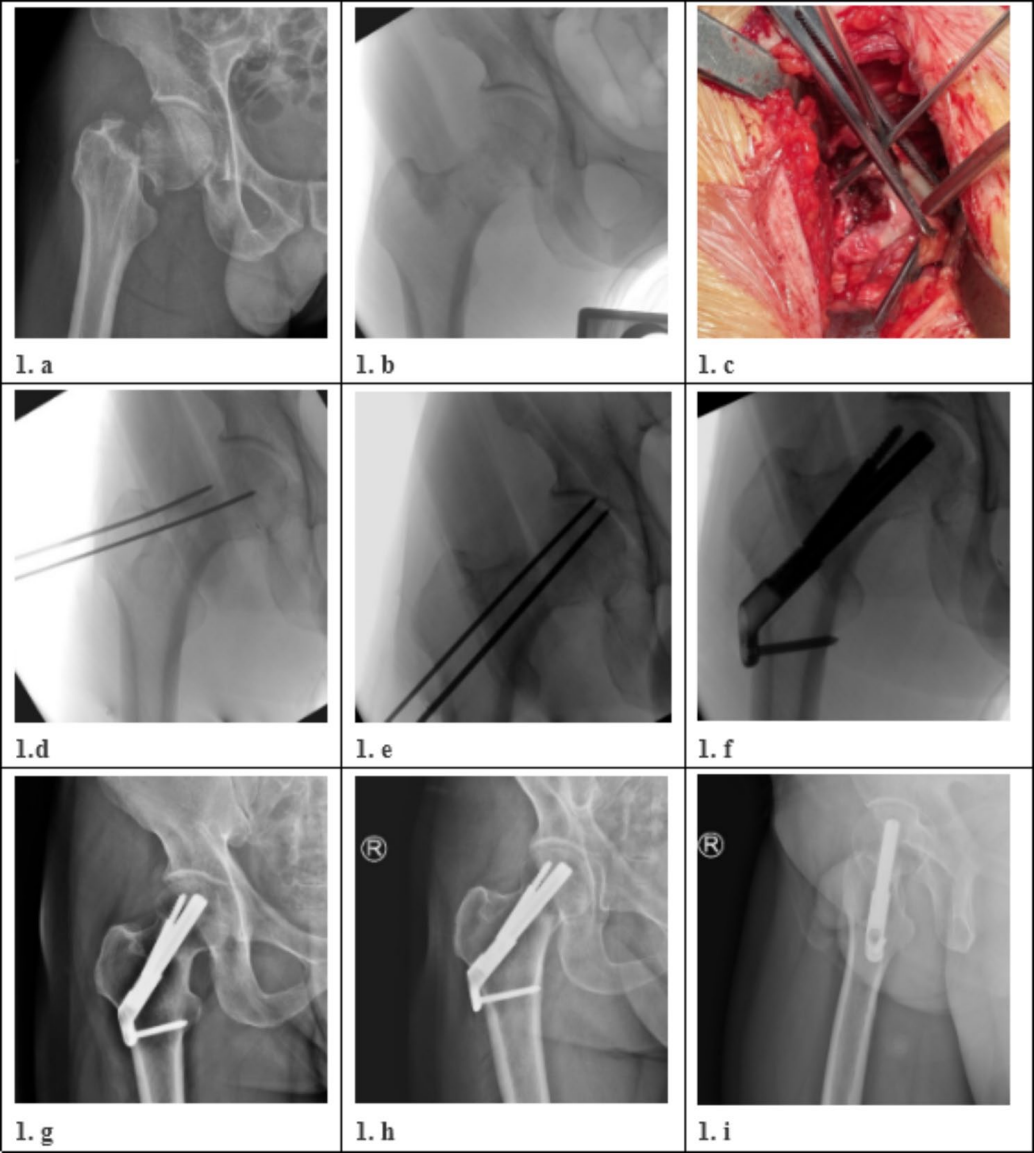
Patient1, Male, fall injury, (1a: Right femoral neck fracture on preoperative X-ray, Garden type IV; 1b: Intraoperative radiography showed that closed reduction could not achieve satisfactory reduction; 1c: Intraoperative incisions image showed that Kirschner wires were inserted into both distal and proximal parts of the fracture to assist reduction; 1d: Intraoperative X-ray showed an anatomic reduction of the fracture; 1e: Intraoperative X-ray showed the insertion of FNS guide needle and an anticockerill needle; 1f: Intraoperative X-ray showed fractures were fixed using FNS and reaching Graden grade I reduction; 1 g: X-ray showed fracture union at 6 months follow-up. 1h1i: At 1 year follow-up, X-ray showed good fracture healing and no ischemic necrosis of femoral head occurred.)
